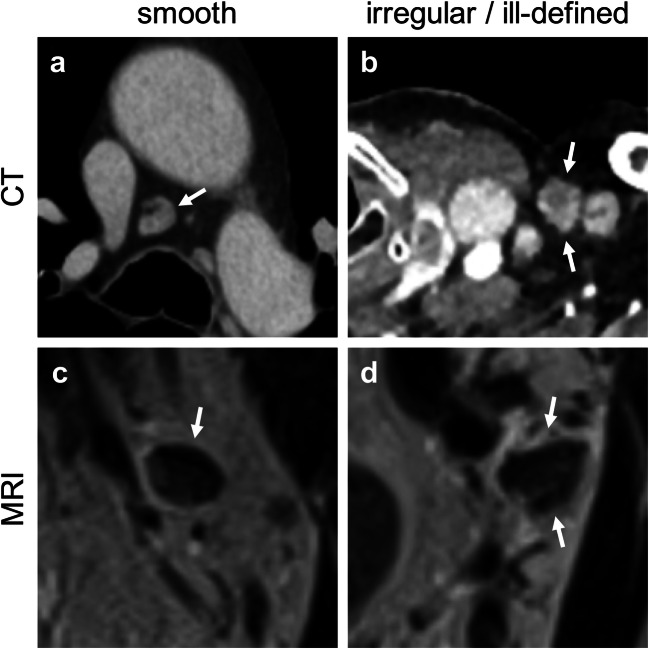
a–d Representative images for criterion “configuration,” feature “border.” a Middle mediastinum, axial, contrast-enhanced (venous phase); b medial supraclavicular on the left side, axial, contrast-enhanced (split-bolus), c obturator on the left side, coronal, T2 weighted; d obturator on the left side, coronal, T2 weightedThe third category “shape” covers two features, the geometric shape and the delineation of the fatty hilum. Both features are usually well assessed on high-spatial resolution CT or MRI but are unspecific so that the maximum of 1 point can be assigned: 0 points (“any shape with preserved fatty hilum”) or (“kidney-bean-like or oval without fatty hilum”) 1 point (“spherical without fatty hilum”) (Fig. 5).
